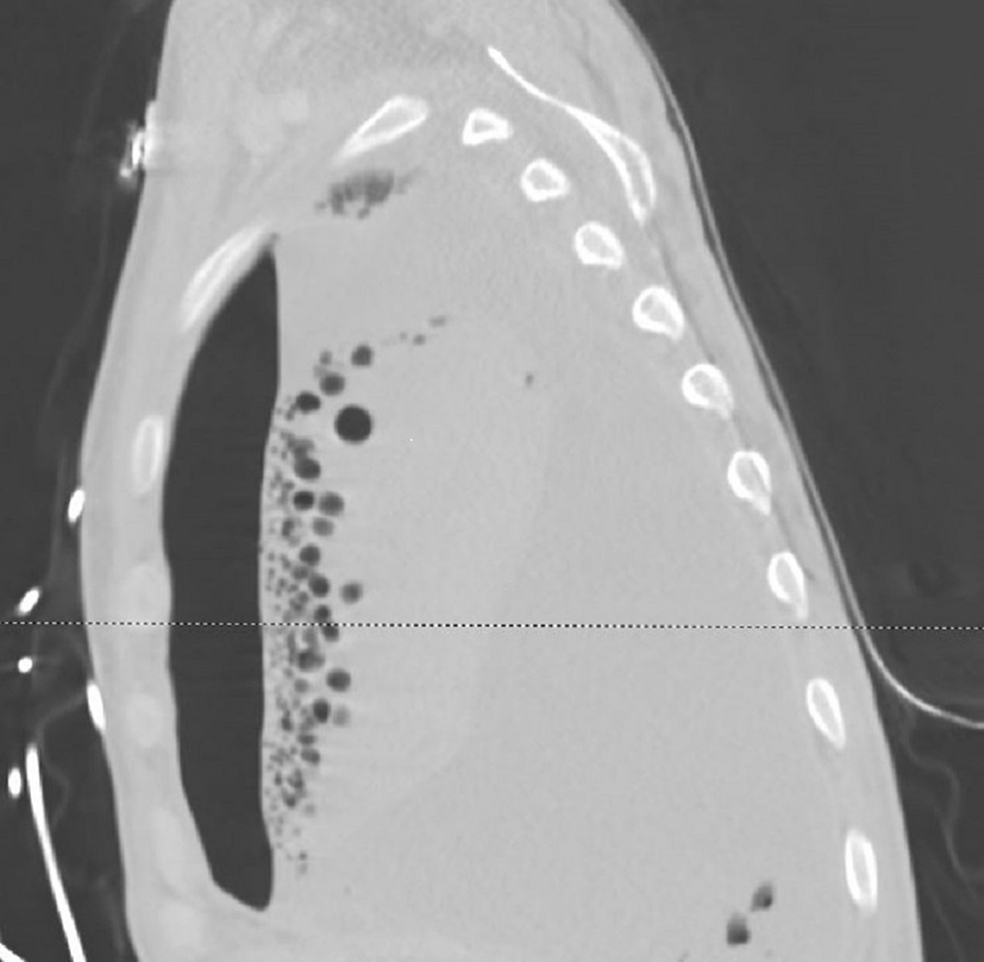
Anteroposterior (AP) view of CT chest showing focal areas of fluid within the pulmonary parenchyma consistent with intraparenchymal abscesses in the right lung
